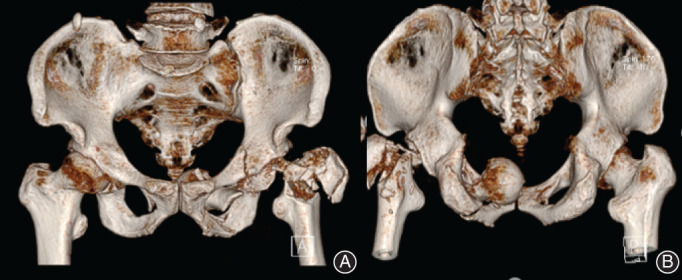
Three‐dimensional reconstruction of CT showing obturator dislocation of the hip joint with greater trochanter fracture.
